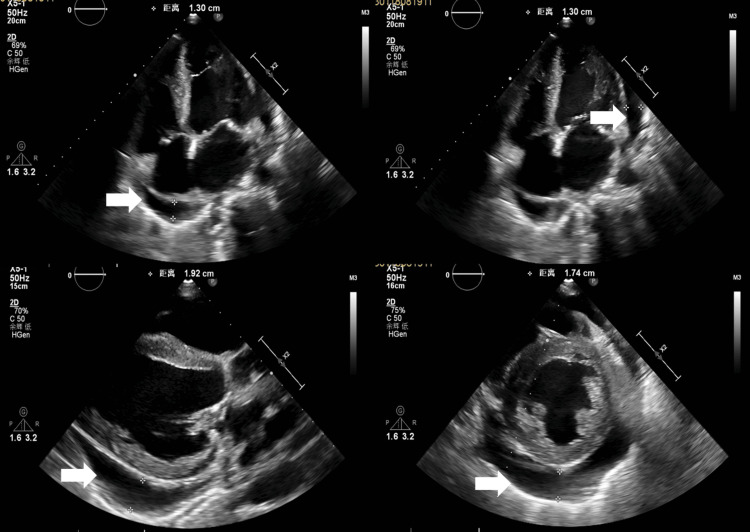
Echocardiographic Visualization of Moderate Pericardial Effusion Cardiac color Doppler ultrasound demonstrates a moderate pericardial effusion. The fluid accumulation is clearly visualized in the standard echocardiographic views, including the apical four-chamber view, parasternal long-axis view, and parasternal short-axis view.
